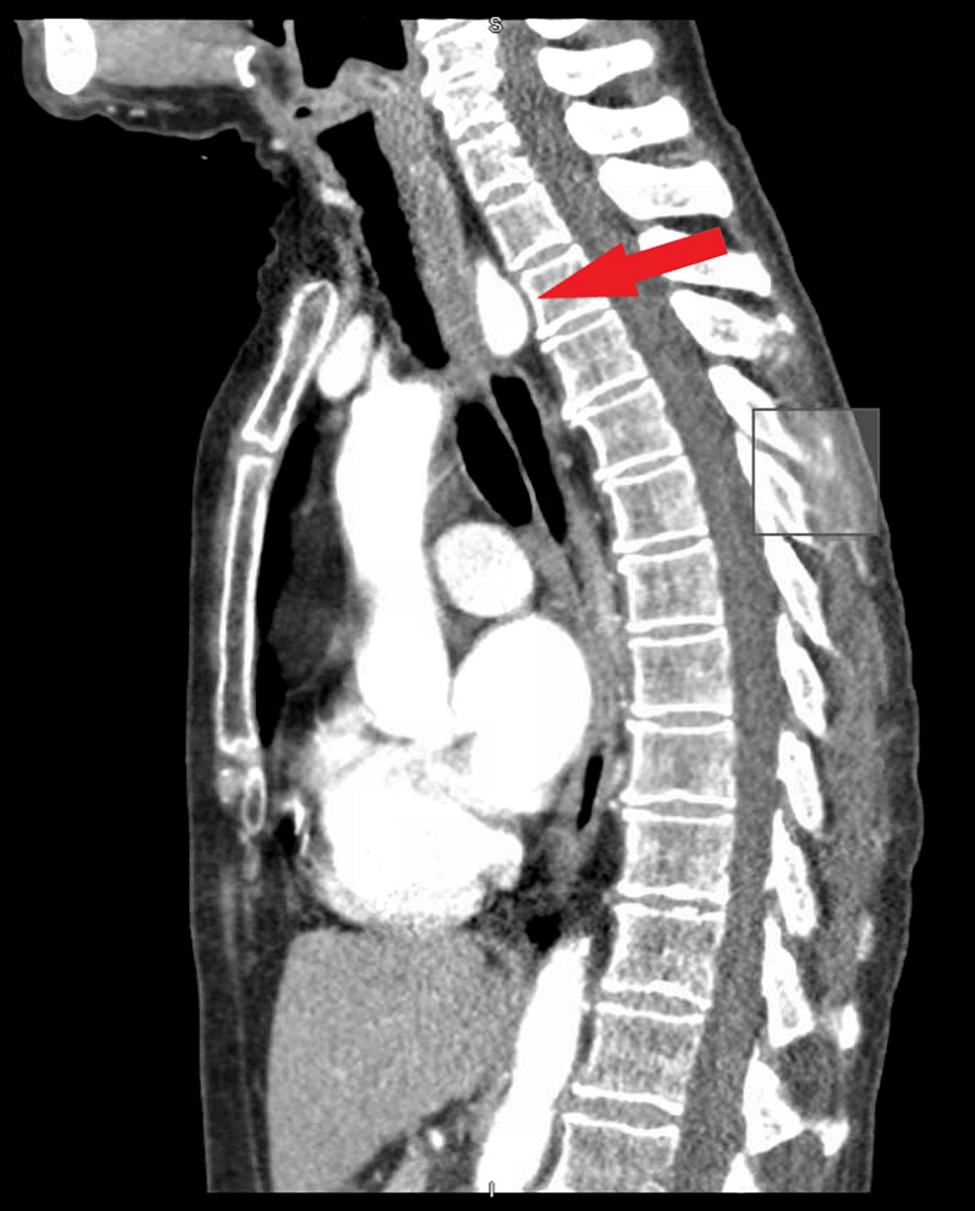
CT neck showing Kommerell diverticula
